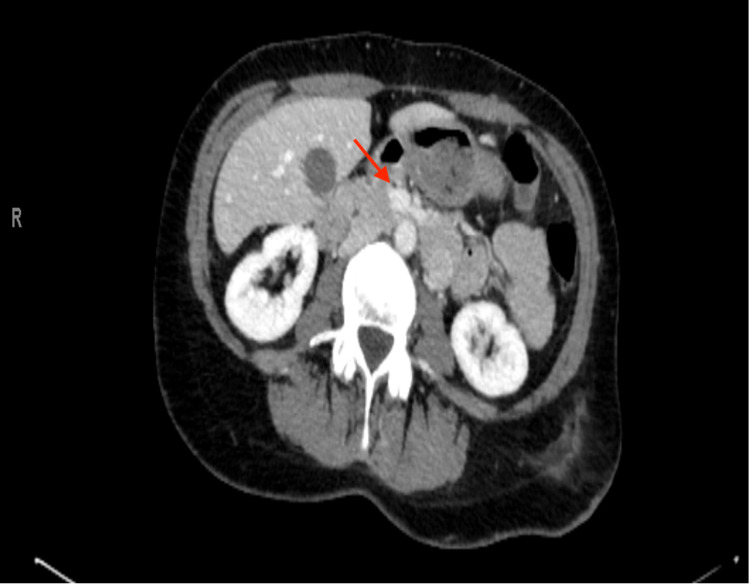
CT abdomen with IV contrast: A round partially exophytic avidly enhancing mass measuring 1.6x1.5cm in the 4th portion of the duodenum, favoured to represent a GIST. GIST - Gastrointestinal Stromal Tumor
